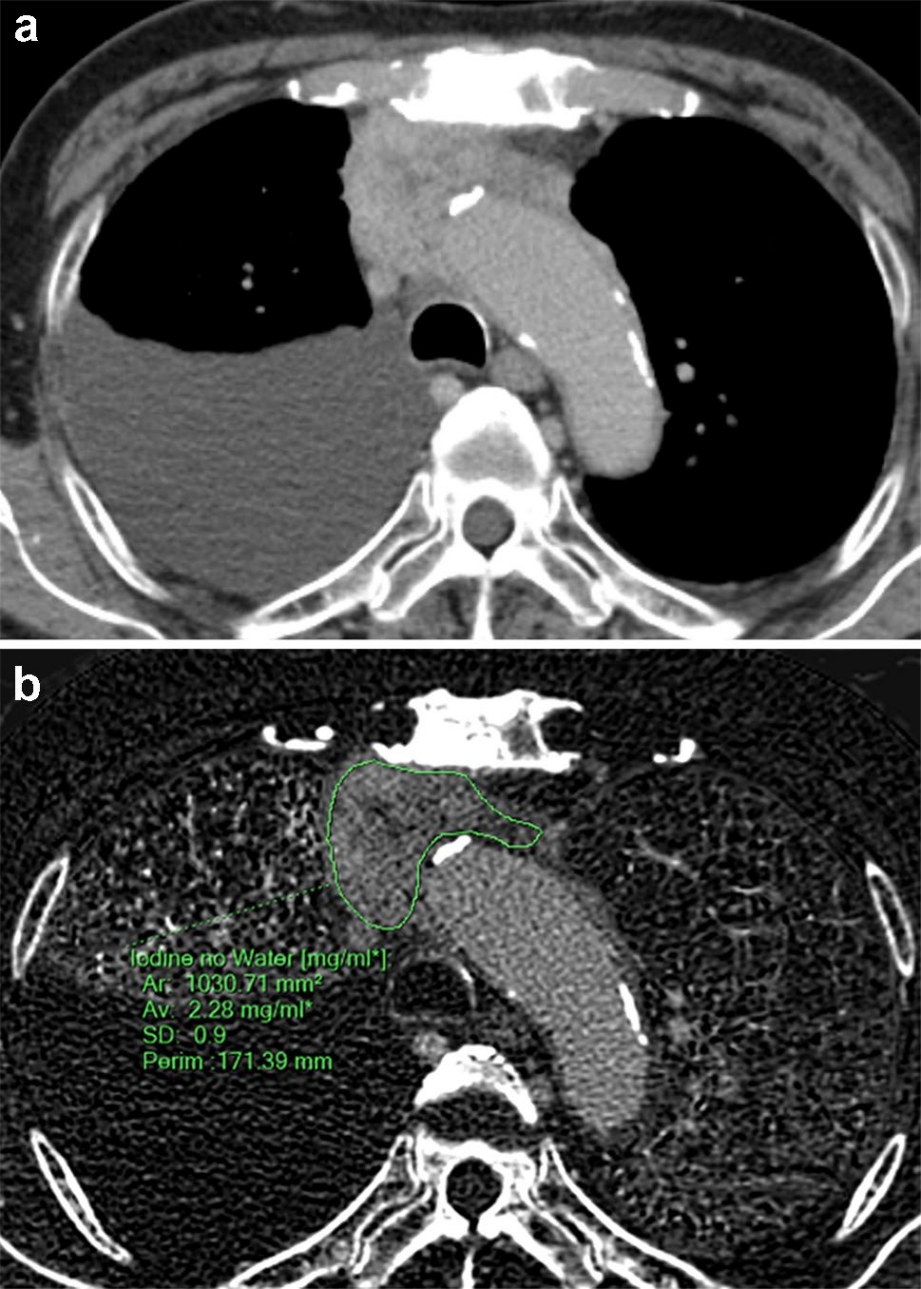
An 89-year-old male with thymic carcinoma. Contrast enhanced CT (a) shows a heterogeneously enhancing, irregularly shaped and ill-defined tumor in the anterior mediastinum, with pleural effusion. Iodine concentration during the equilibrium phase (b) and extracellular volume fraction are 2.28 mg/mL and 40.8%, respectively
